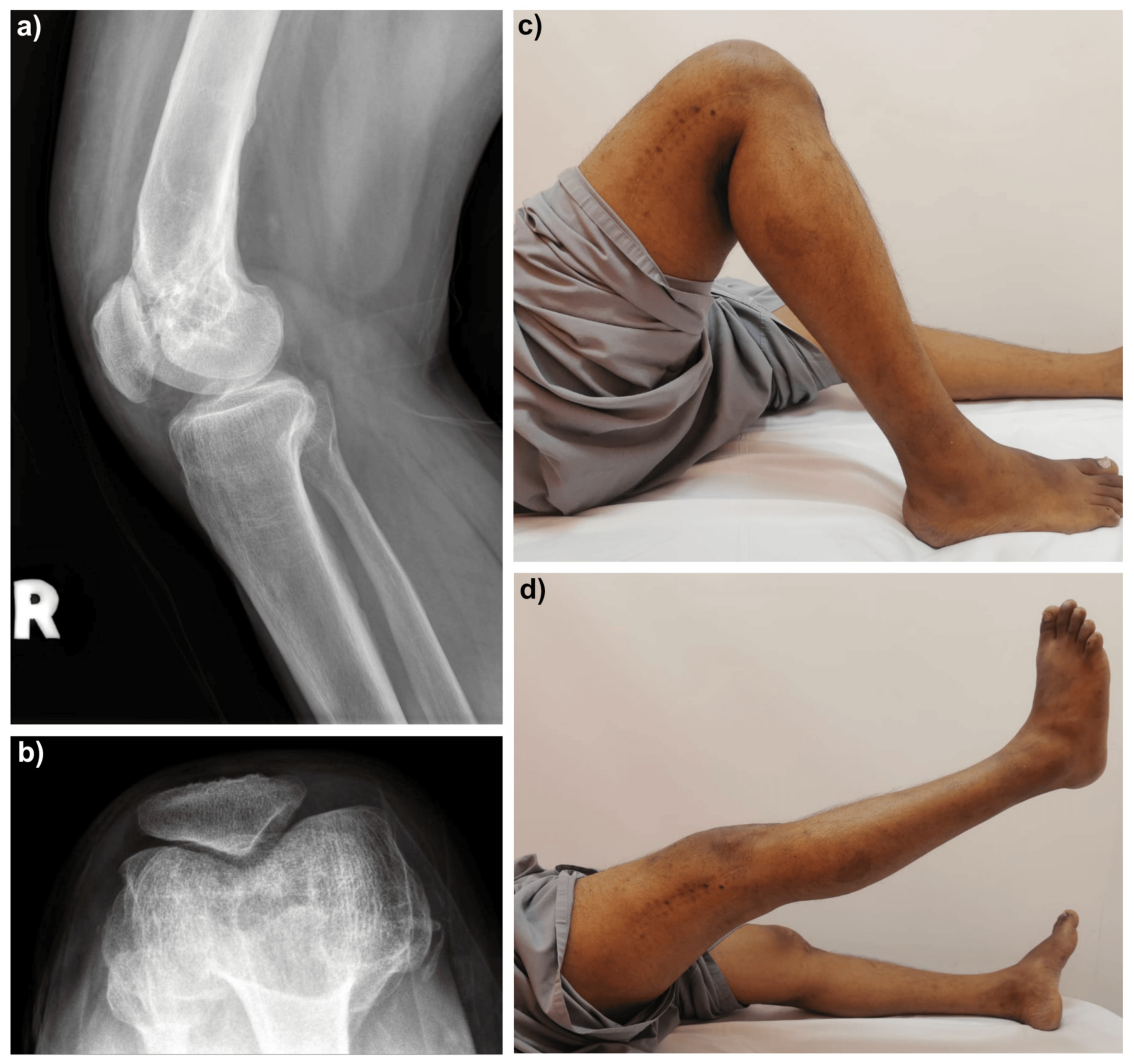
Two-year post-operative radiographic and clinical assessment of the right knee (a) Lateral radiograph showing restoration of patellofemoral joint alignment
